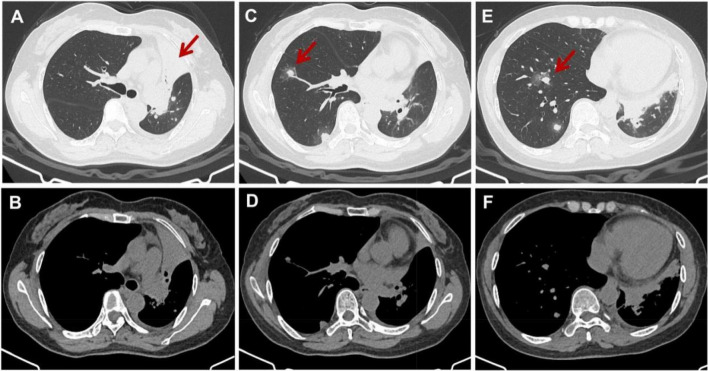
Computed tomography of the chest at admission. Images reveal (A) consolidation of the left upper lobe with atelectasis (red arrow), as well as (C,E) multiple bilateral patchy opacities, nodules, and masses with a peripheral halo sign (red arrows). The corresponding mediastinal images are shown in the lower row. (B,D,F) Represent the mediastinal window images corresponding to the layers of panels (A,C,E) respectively.
